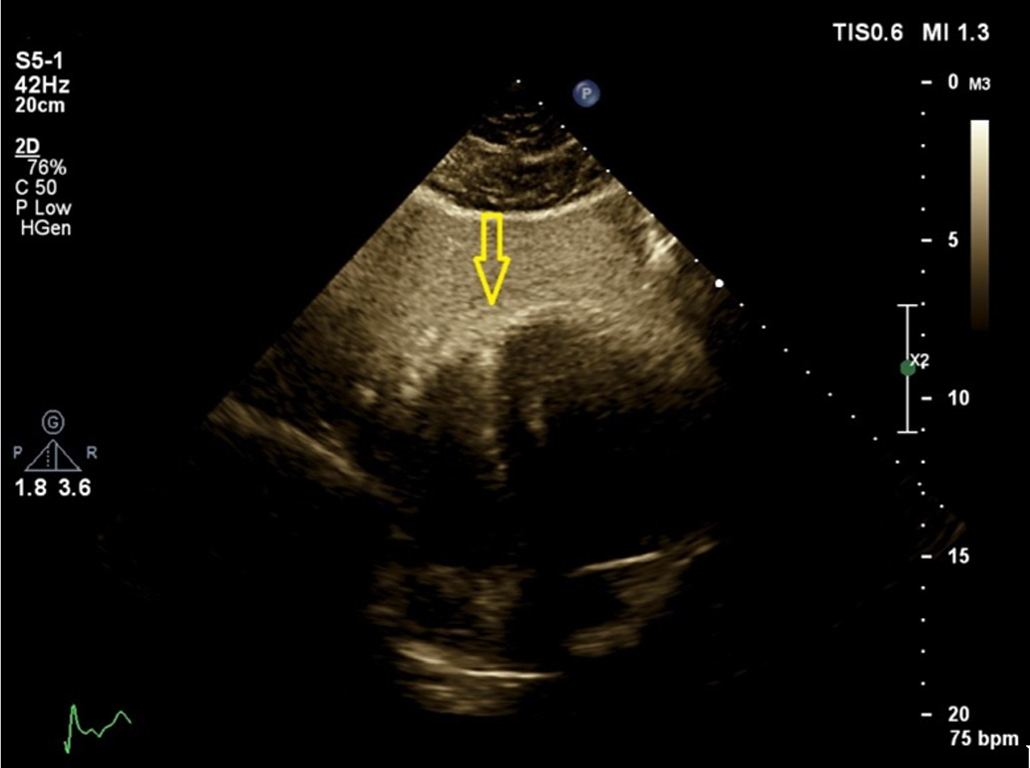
Mesocardia in the subcostal view in transthoracic echocardiography. The arrow shows the cardiac apex, which is in the midline and is suggestive of mesocardia.
